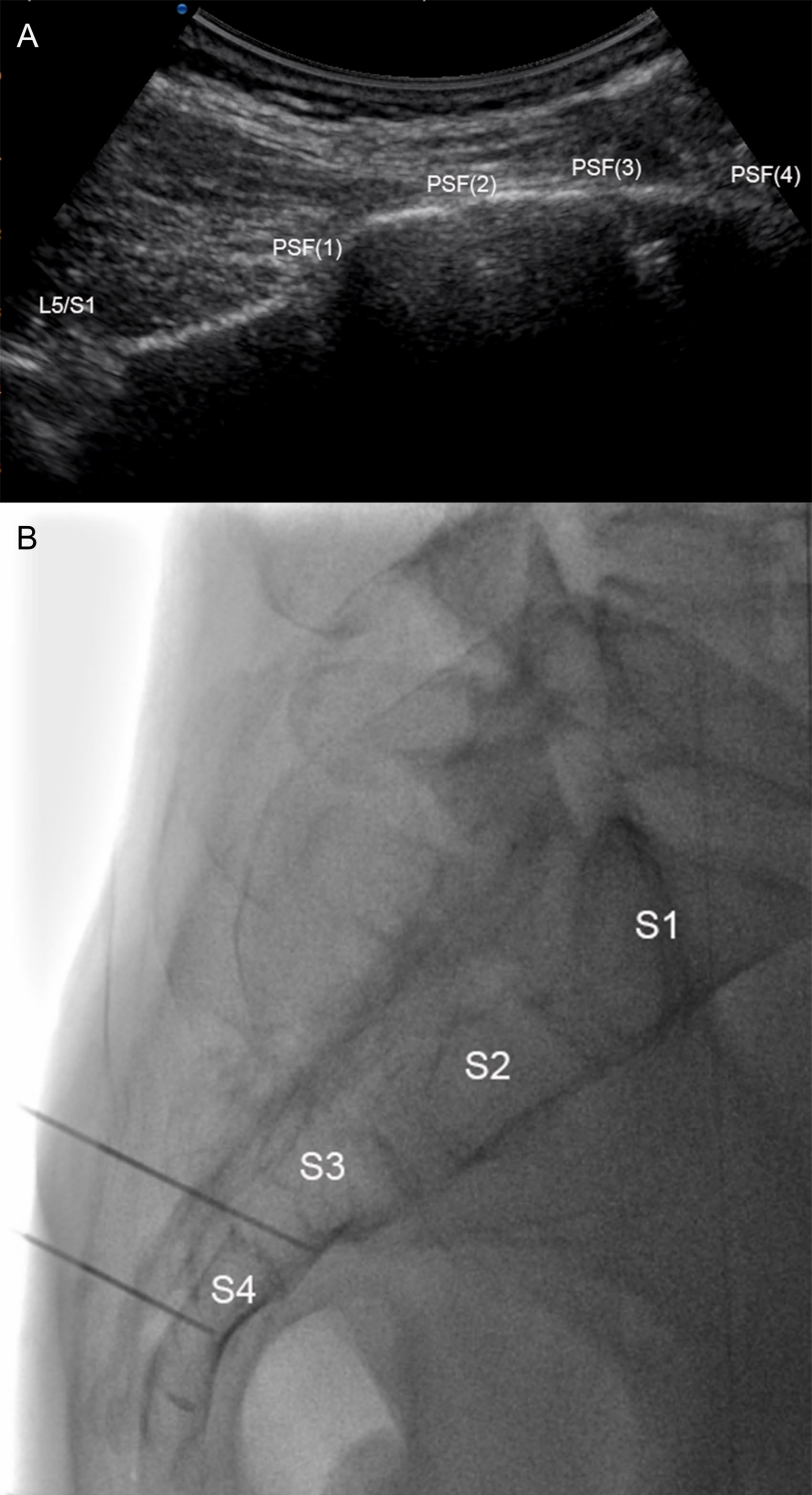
Ultrasound combined with DSA-guided pulsed radiofrequency for perineal herpes zoster. (A) Longitudinal ultrasound view of the posterior sacral foramen. (B) X-ray in the lateral position. The radiofrequency needle passed through the sacral foramen (S3, 4), with the tip of the needle located in front of the sacral foramen. PSF: posterior sacral foramina.
